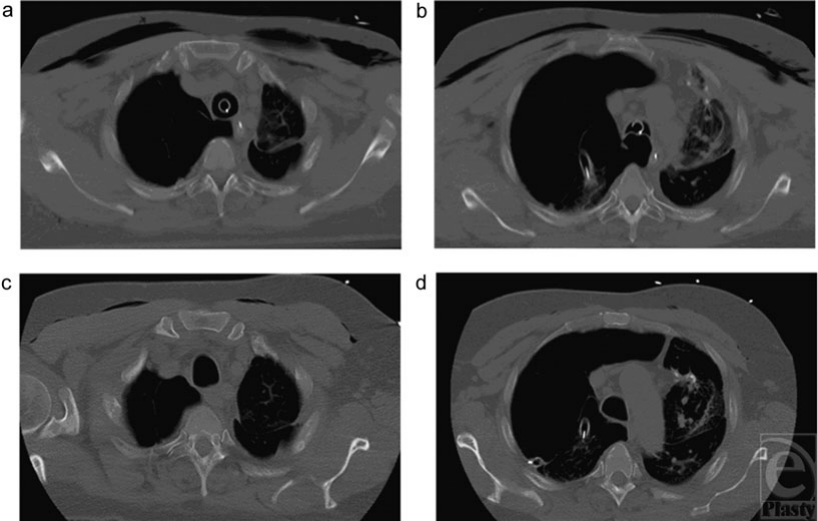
Representative saggital computed tomography scan images of the chest at the level of the sternoclavicular joint (*a*) and the top of the aortic arch (*b*)taken shortly after tube thoracostomy. Note the extensive bilateral subcutaneous emphysema. Corresponding sections after 48 hours of NPWT (*c*, *d*, respectively) demonstrate near-complete resolution of SE.
